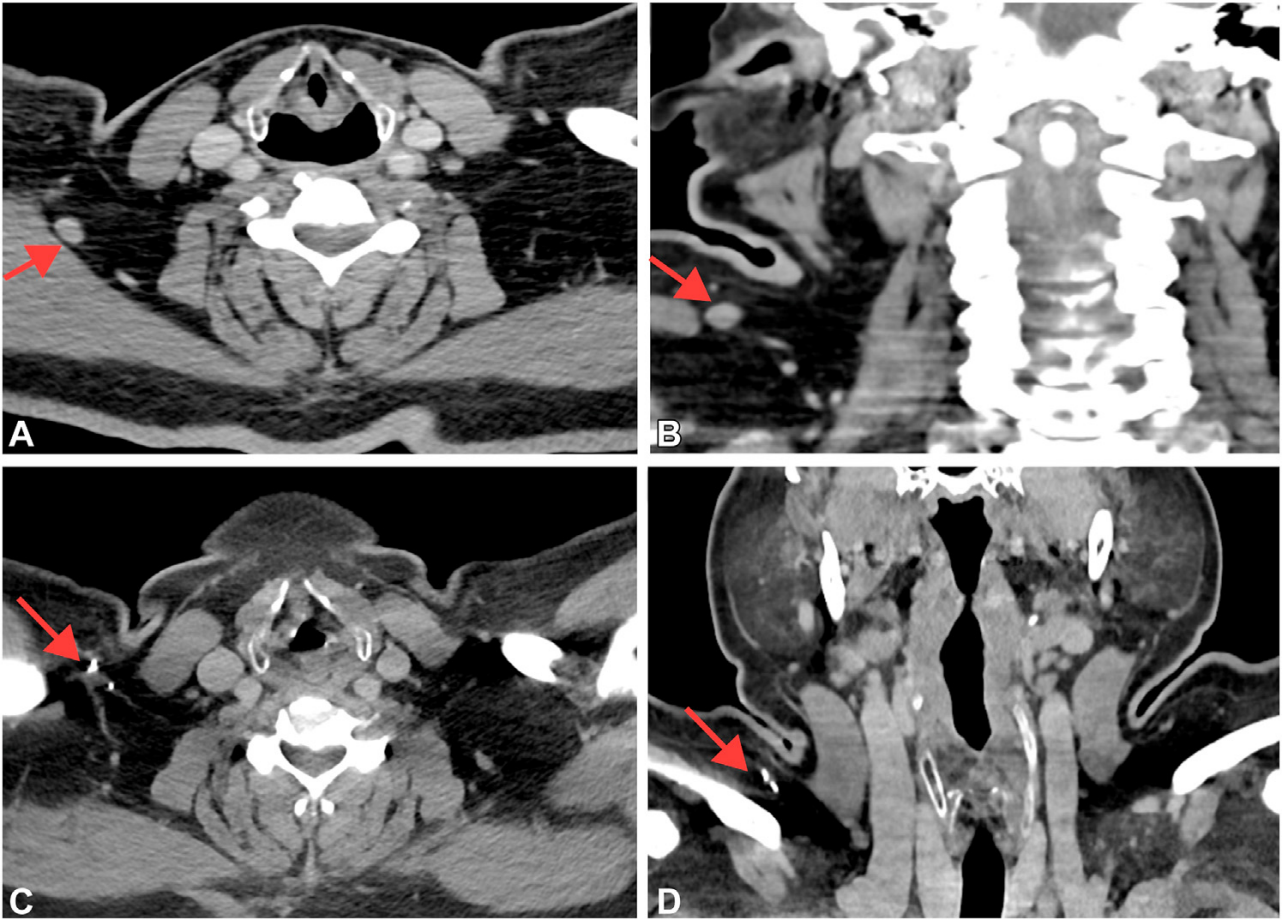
Radiologic evaluation of the lesion before and after treatment. Axial (A) and coronal (B) images after one month of pembrolizumab treatment demonstrates a new prominent 8 mm short axis right supraclavicular lymph node slightly posteroinferior to the original 13 mm right supraclavicular lymph node (*red arrows*), suggesting additional nodal metastases. Axial (C) and coronal (D) images of the neck from the patient's contrast-enhanced computed tomography (CT) one year later post Pembrolizumab treatment continues to show complete remission without evidence of disease recurrence or metastatic disease. Contrast-enhanced CT of the chest, abdomen, and pelvis (not shown) also similarly showed no evidence for metastatic disease.
